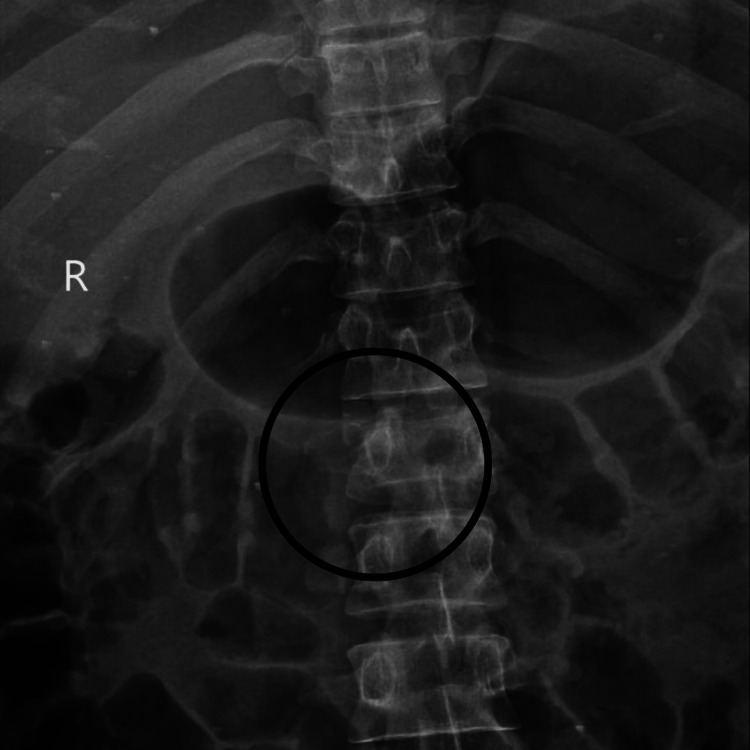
X-ray of spine showing L1 vertebra fracture The circle shows acute wedge compression fracture with retropulsion of the L1 vertebra.
